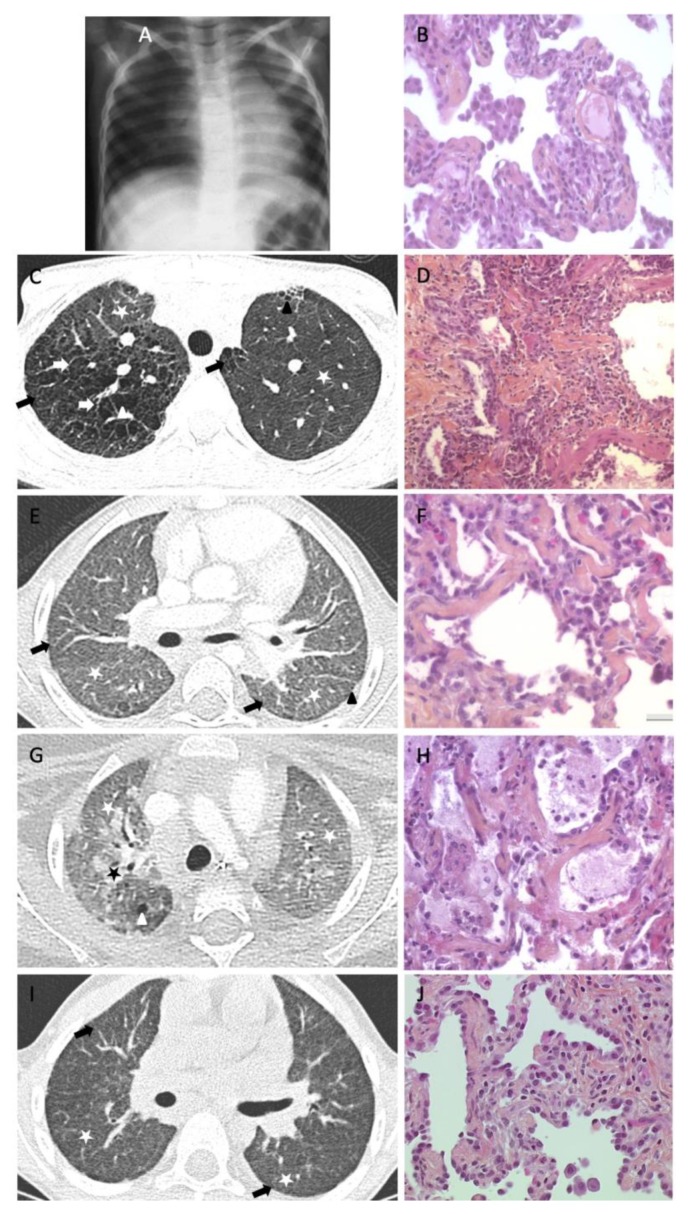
Pulmonary fibrosis examples in children. Panels A and B: Patient 1. (A) Chest X-ray: diffuse repartition of ground-glass opacities (GGOs). (B) Lung biopsy at age 6: no or mild parenchymal distortion, diffuse thickening of the alveolar walls, hyperplasic alveolar epithelial cells (AECs), inflammatory cell recruitment, mild fibroblasts activation, and mild collagen deposition. Panels C and D: Patient 2. (C) Transverse HRCT scan obtained at the level of the upper lobes: diffuse repartition of mild GGOs (white stars), reticulations (with interlobular septal thickening (white arrows) and intralobular lines (black arrows)), and numerous cystic lesions (white arrowheads) with focal left subpleural honeycombing (black arrowhead). Reticulations and cystic lesions are wider on the right. (D) Lung biopsy: parenchymal distortion, inflammatory cell recruitment, lymphoid nodules, and mild collagen and severe elastic fiber deposition. Panels E and F: Patient 3. (E) Transverse HRCT scan obtained under the level of the carina: diffuse repartition of GGOs (white stars), moderate reticulations (with intralobular lines (black arrows)), and few subpleural cystic lesions (black arrowhead). (F) Lung autopsy: no parenchymal distortion, diffuse thickening of the alveolar walls, hyperplasic AECs, mild inflammatory cell recruitment, and elastic fiber deposition. Panels G and H: Patient 4. (G) Transverse HRCT scan obtained at the level of the upper lobes: diffuse repartition of severe GGOs (white stars), consolidations (black star), and few cystic lesions (white arrowhead). (H) Postmortem biopsy: no parenchymal distortion, diffuse thickening of the alveolar walls, hyperplasic AECs, mild inflammatory cell recruitment, elastic fiber deposition, and moderate alveolar proteinosis: intra-alveolar deposit with giant cells and liproproteic material. Panels I and J: Patient 5. (I) HRCT scan obtained at the level of the lung bases: diffuse and homogeneous repartition of GGOs (white stars) with reticulations (with intralobular lines (black arrows)). (J) Lung biopsy: no parenchymal distortion, diffuse thickening of the alveolar walls, hyperplasic AECs, and moderate inflammatory cell recruitment.
